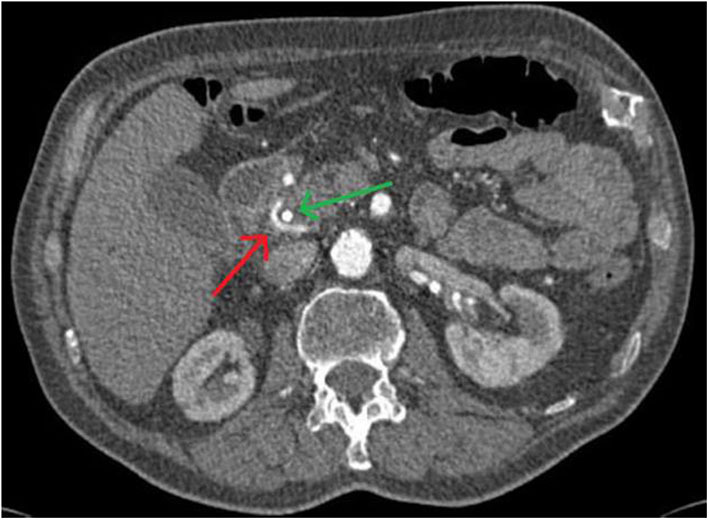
The Bühler anastomosis can be surmised in the axial projection of the CT scan. The red arrow points to the vascular variant, the green one to the stent in the hepatic duct.
